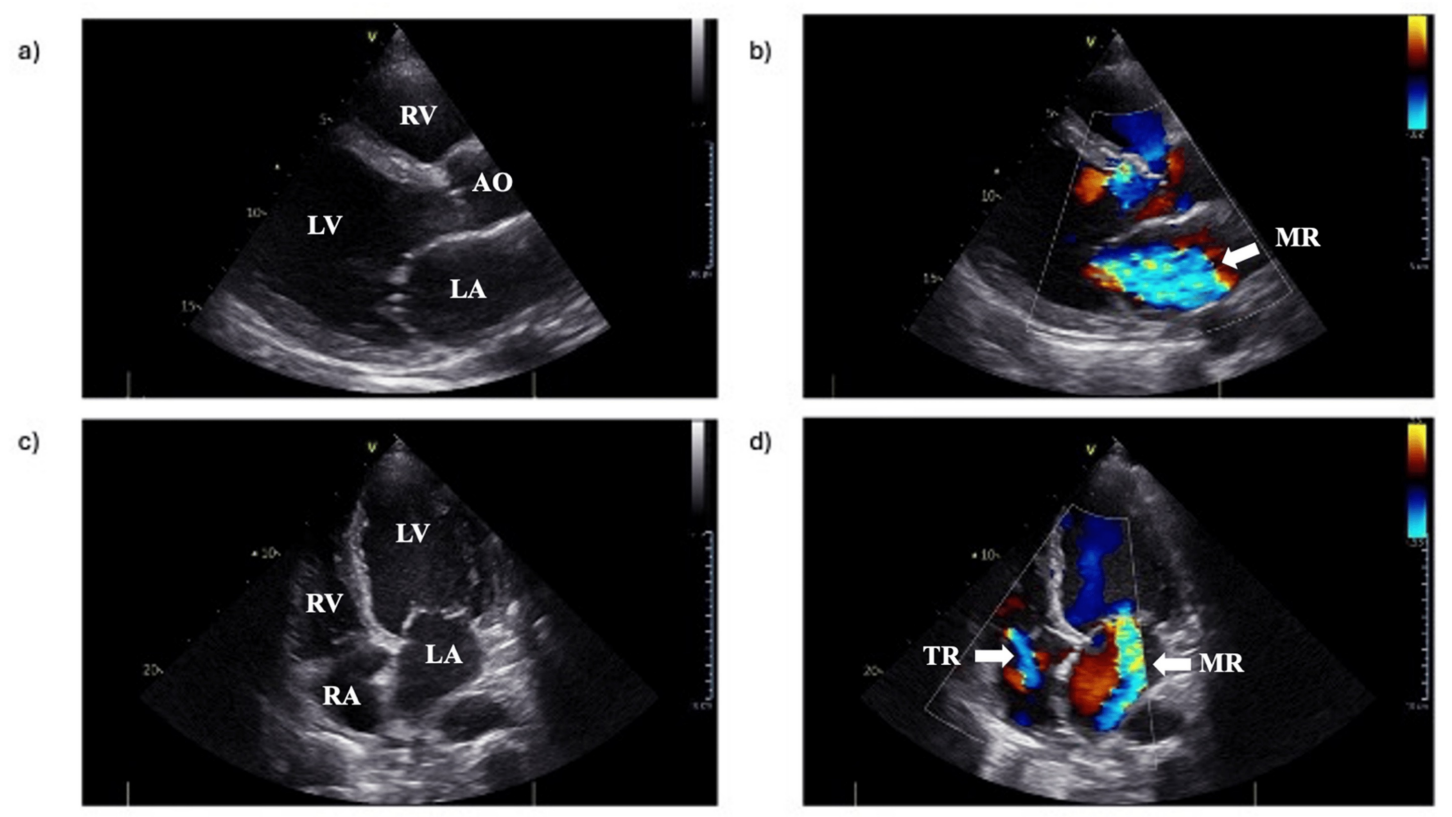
Transthoracic echocardiogram at admission showing a severely dilated left ventricle with severe mitral regurgitation and moderate tricuspid regurgitation. a) PLAX view. b) Color flow Doppler imaging on the PLAX view. c) AP4CH view. d) Color flow Doppler imaging on the AP4CH view. AO, aorta; AP4CH, apical four-chamber; LA, left atrium; LV, left ventricle; MR, mitral regurgitation; PLAX, parasternal long axis; RA, right atrium; RV, right ventricle; TR, tricuspid regurgitation
